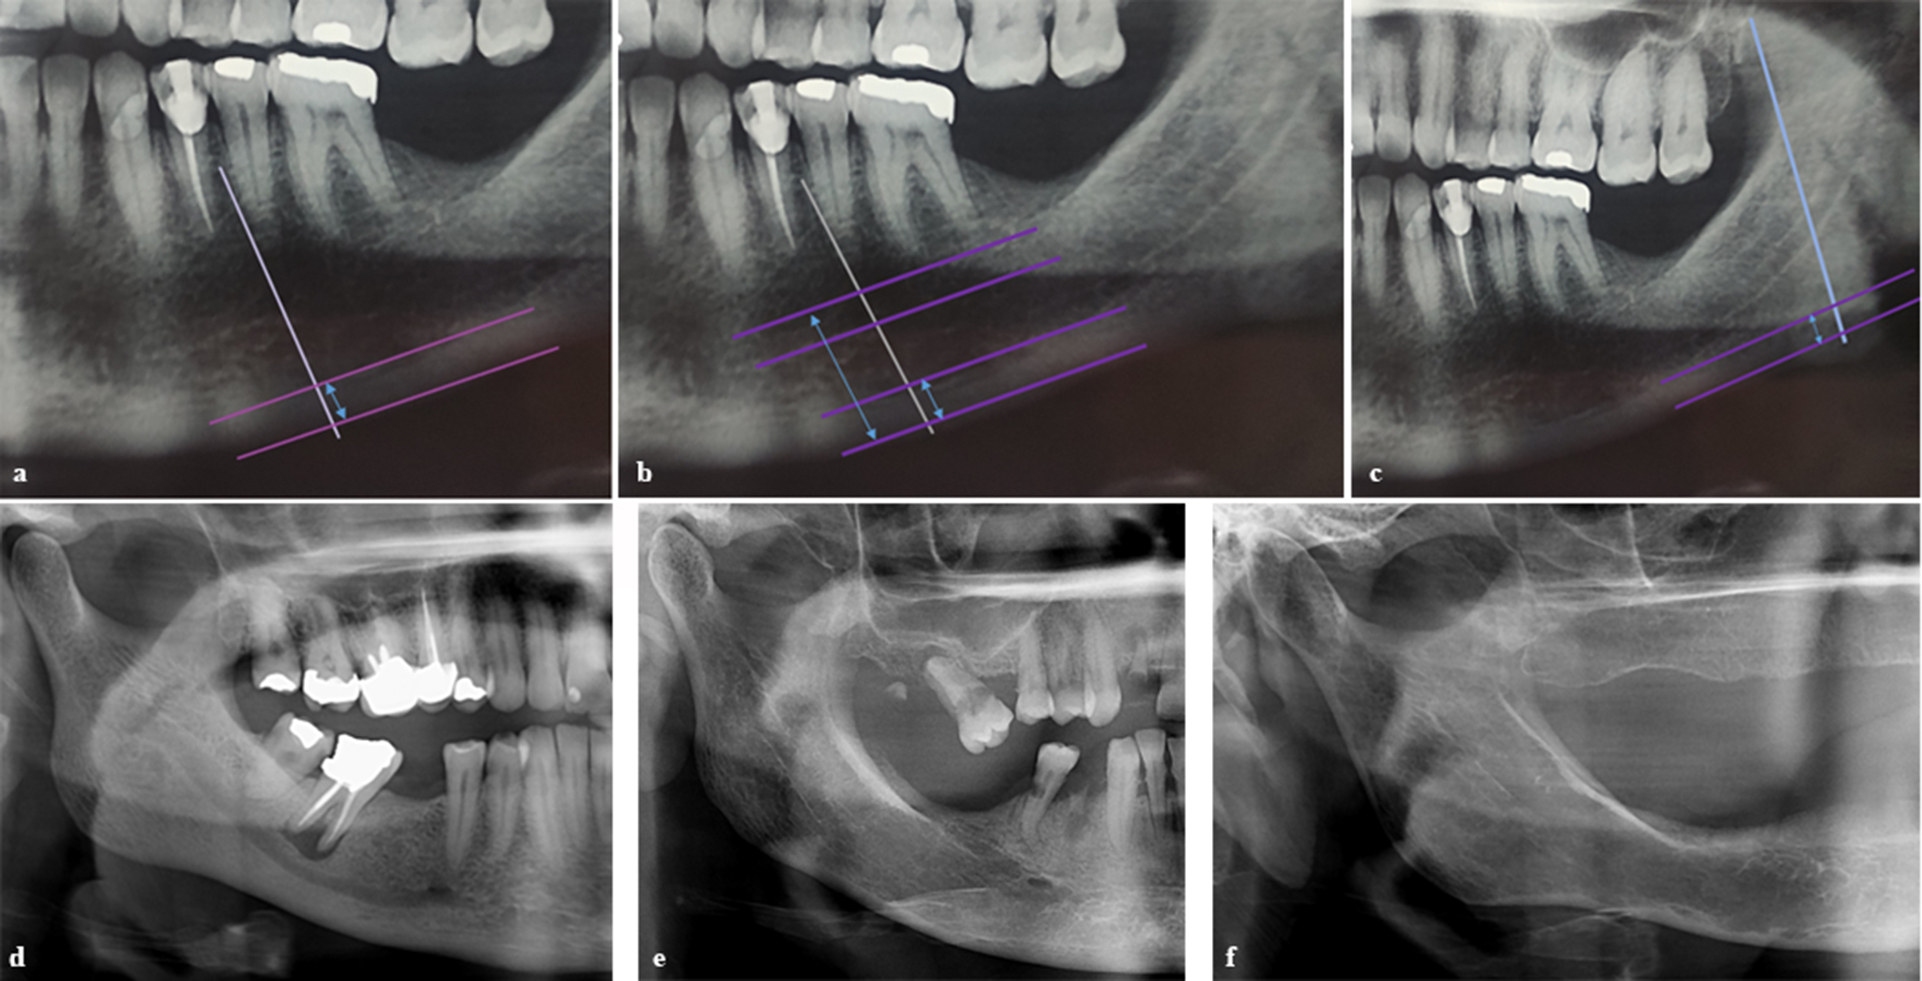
Analyzed indexes in panoramic radiographs: (a) mental index, (b) panoramic mandibular index, (c) antegonial index, (d) mandibular cortical index class 1 and dense trabecular pattern, (e) mandibular cortical index class 2 and heterogenous trabecular pattern, and (f) mandibular cortical index class 3 and sparse trabecular pattern
